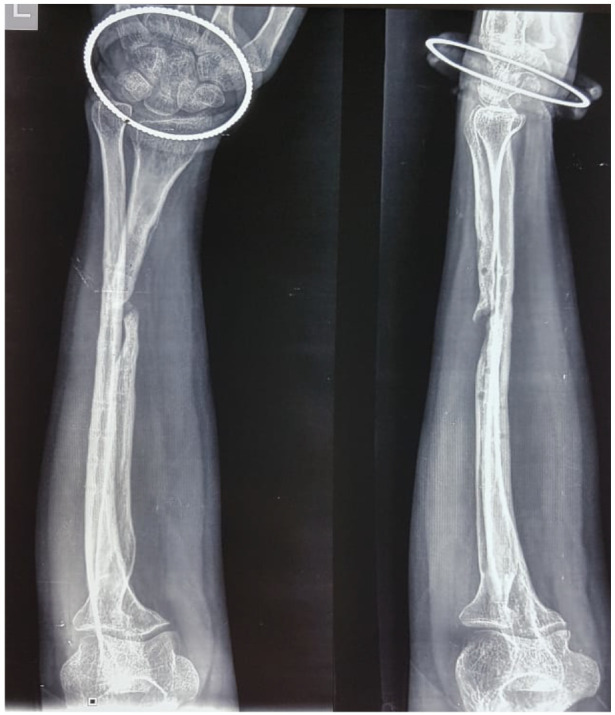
Initial preoperative radiograph
